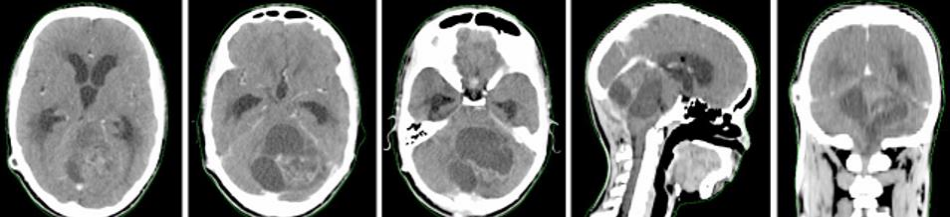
cerebral CT sections with contrast product injection in all three planes, showing the cerebellar tumour process with triventricular hydrocephalus
